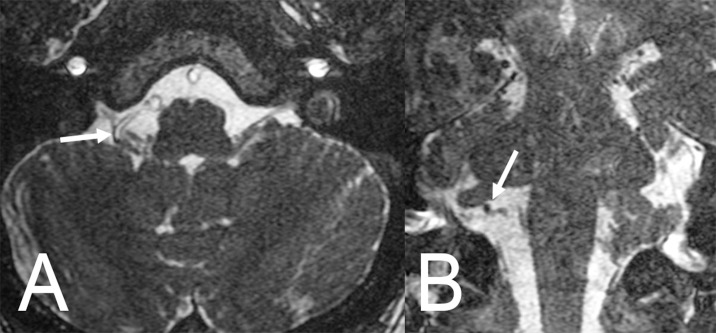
Non-enhanced brain magnetic resonance imaging (MRI). T2 high-resolution (FIESTA) axial (A) and coronal (B) slices showing the vessel involved in the neurovascular compression syndrome in the meatus of the right jugular foramen between the right IX cranial nerve and the posterior and inferior cerebellar artery (arrows).
